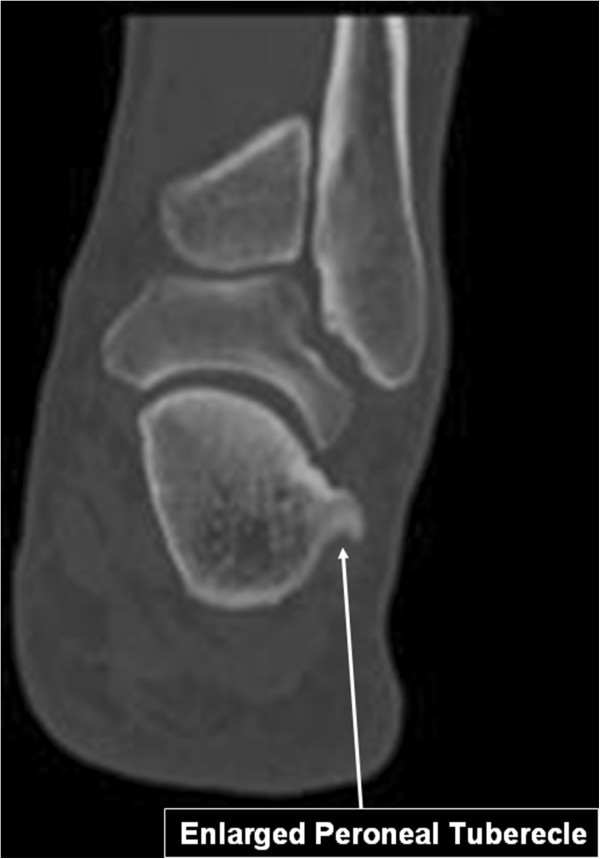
Coronal CT scan view showing the enlarged peroneal tubercle.
